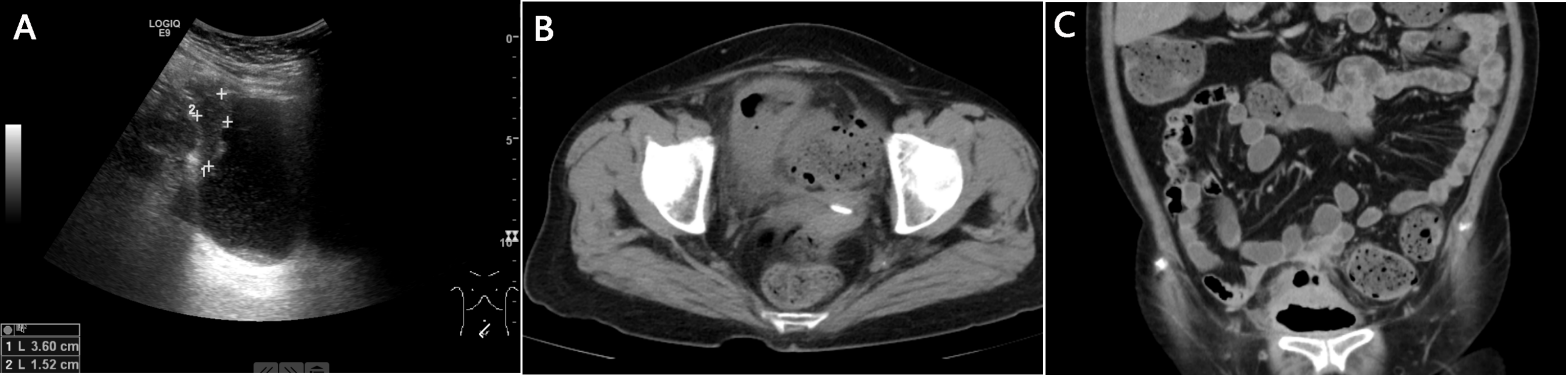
Imaging examinations. (A): Ultrasound examination. The right bladder wall was thickened, and a 3.6 cm × 1.5 cm lesion was revealed; (B): Abdominopelvic computed tomography (CT). Focal thickening of the bladder wall and gas in the bladder were noted; (C): Computed tomography urography (CTU). Gas between the bladder dome and adjacent sigmoid colon, and a thickened bladder and sigmoid colon wall were revealed.
